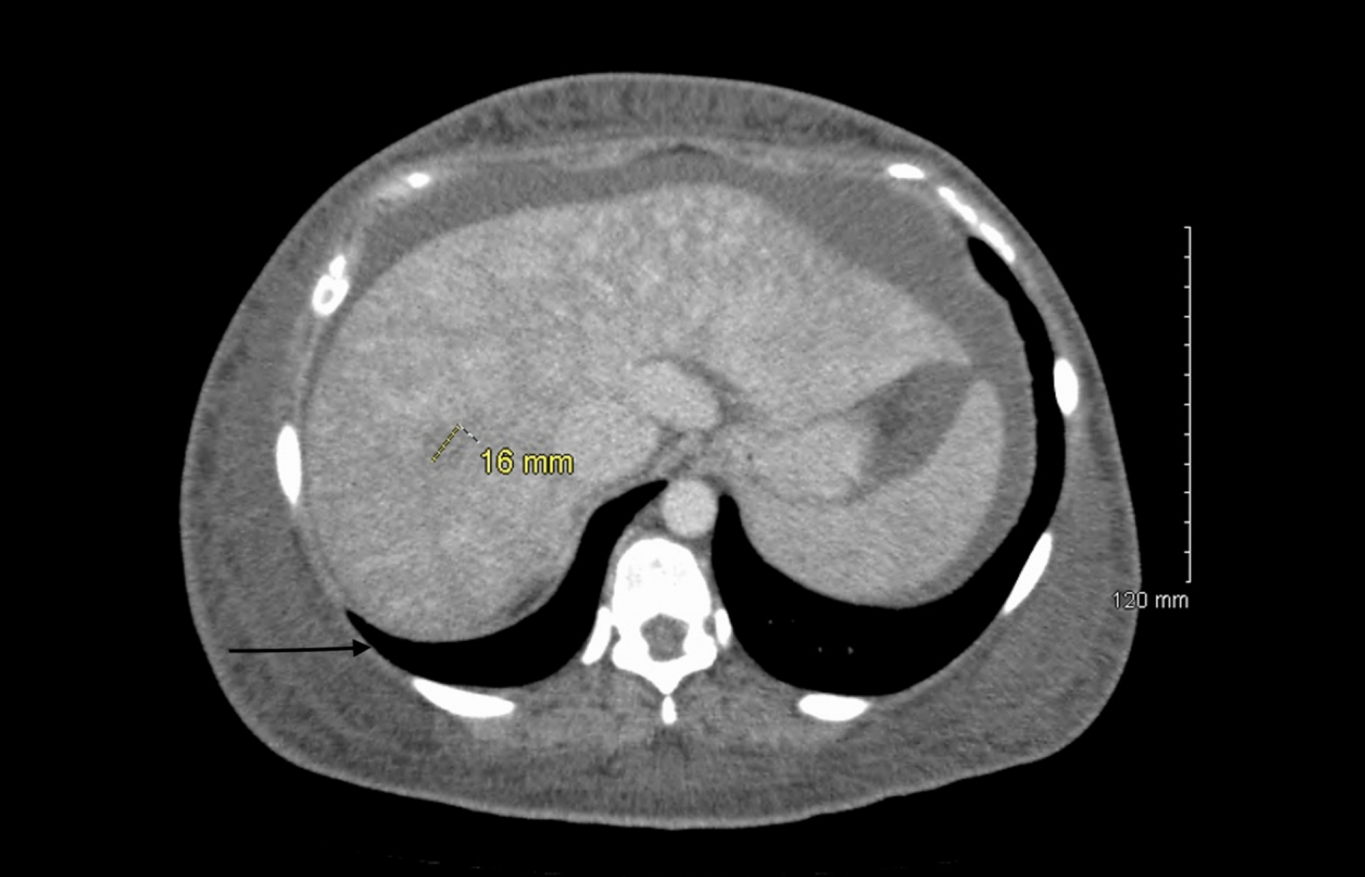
CT scan with the black arrow showing mild abdominal ascites and a 16-mm mass at the junction of segments VII and VIII of the liver, which is suggestive of congestive hepatomegaly.
